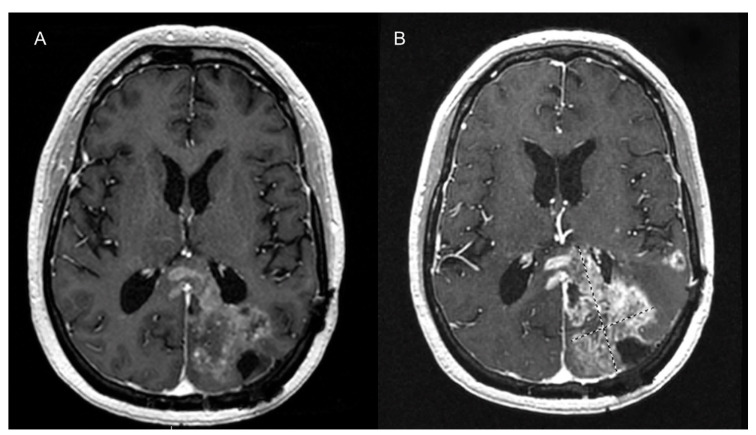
T1-weighted contrast-enhanced axial image of the brain with a left occipital glioblastoma. (A) This image shows enhancing local tumoral recurrence, prior to starting anti-angiogenic therapy. (B) Nine months after initiation of anti-angiogenic therapy shows marked reduction of the enhancing component and associated vasogenic edema.
